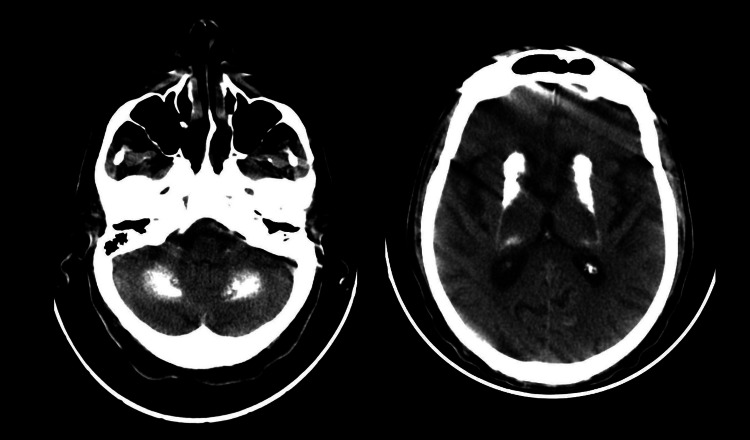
A CT scan of the head without contrast showed dense calcification of the dentate nuclei and the basal ganglia and subcortical calcification of the frontal and occipital lobes
